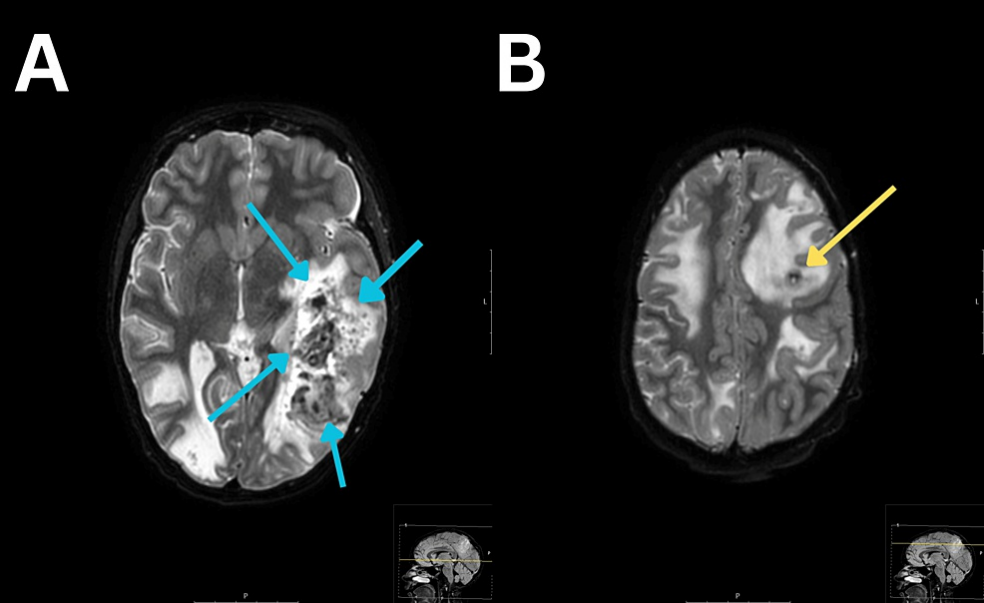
T2-Weighted MRI The blue arrows indicate a hemorrhagic imbibition in the left occipitotemporal region (image A). The yellow arrow shows a smaller hemorrhagic imbibition in the left frontal region (image B). MRI, magnetic resonance imaging.
